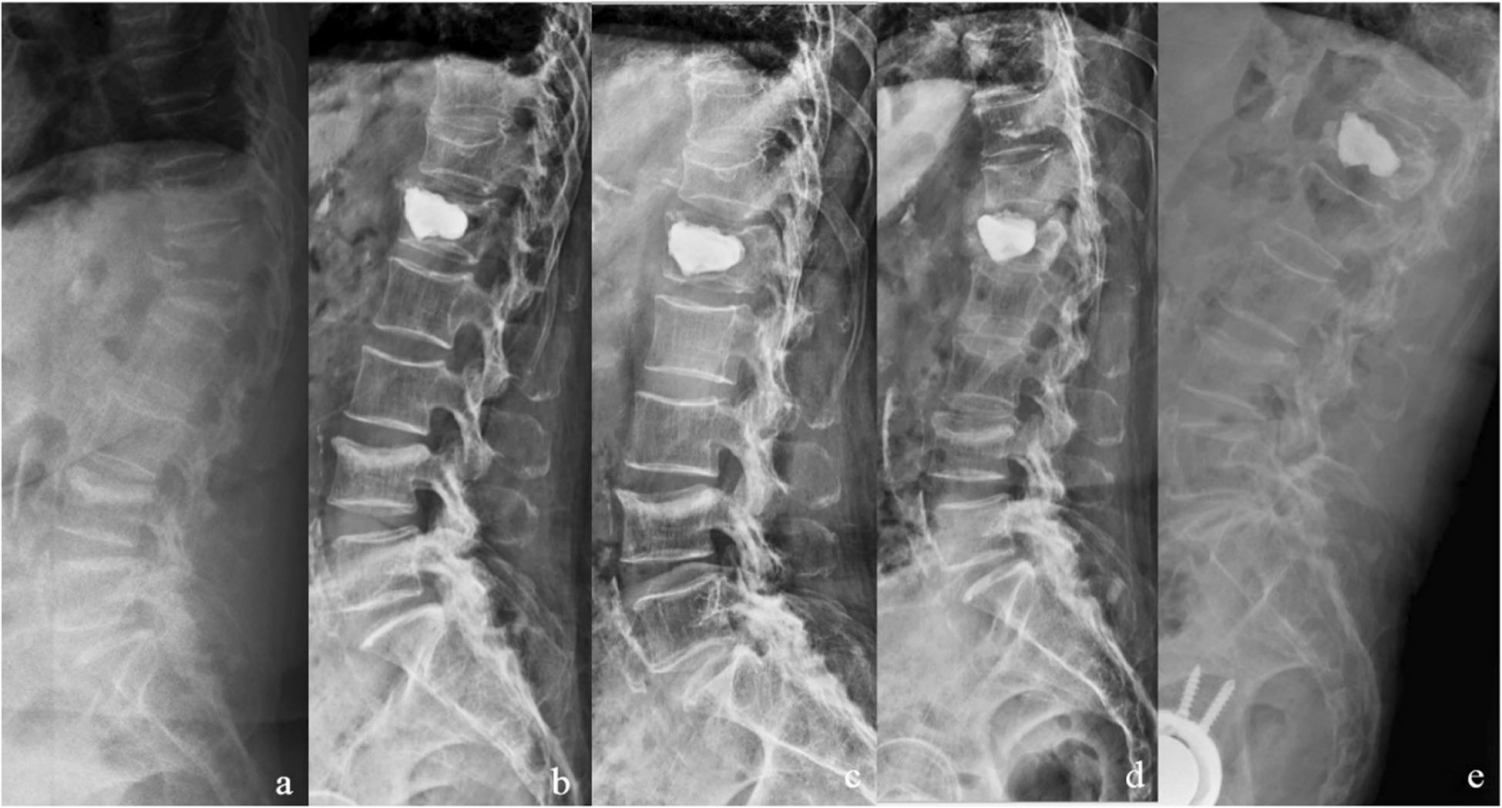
A 73-year-old female patient with osteoporotic compression fracture of L1 vertebral body with kyphosis was treated with PVP alone. a L1 severe compression fracture with kyphosis in preoperative. b Postoperative 3D X-ray showed that the height of L1 vertebra recovered basically and kyphosis was corrected. c–e X-ray showed L1 vertebral was collapsed with kyphosis in 6 months, 12 months, and 24 months follow-up
